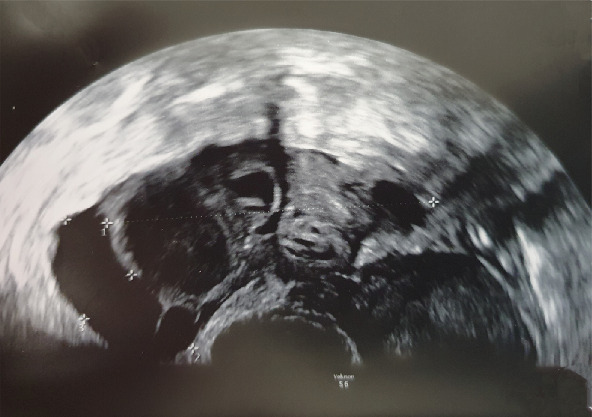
Transvaginal sonography finding in the right adnexal region after the first PID episode.
